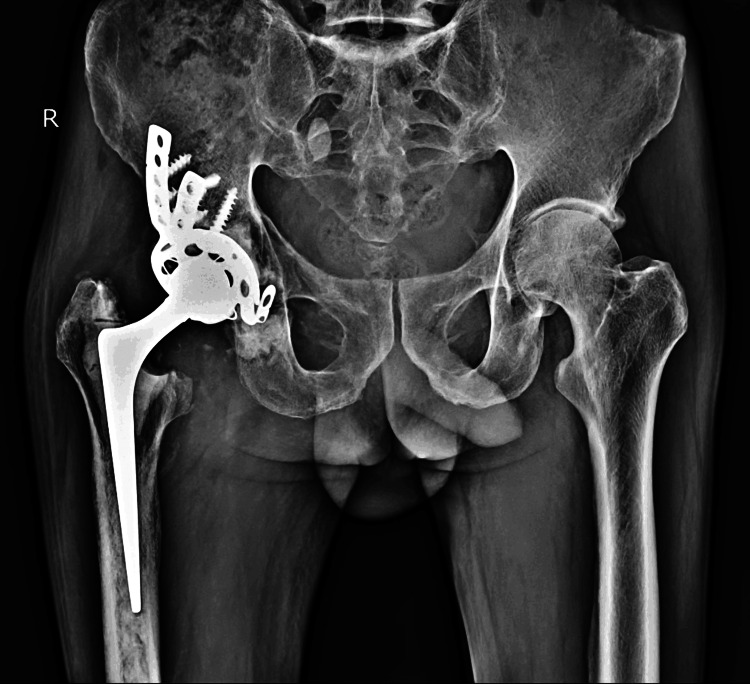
Post-operative X-ray of the pelvis with both hips (anteroposterior view)
